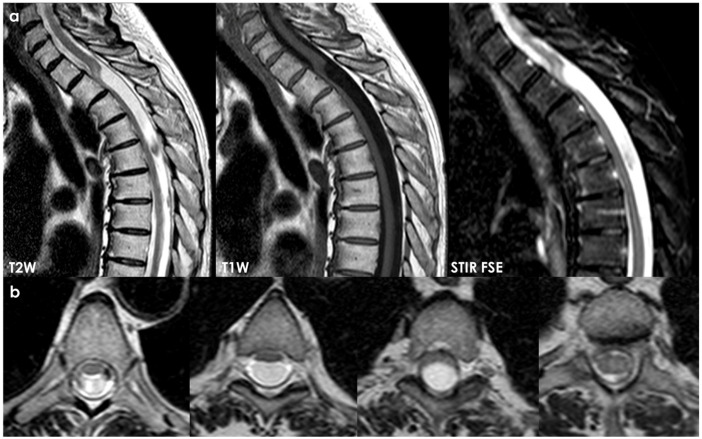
Post-traumatic spinal arachnoid web with scalpel sign and intraspinal lesion. (a) shows sagittal MRI and (b) the axial sections around the attraction level.
